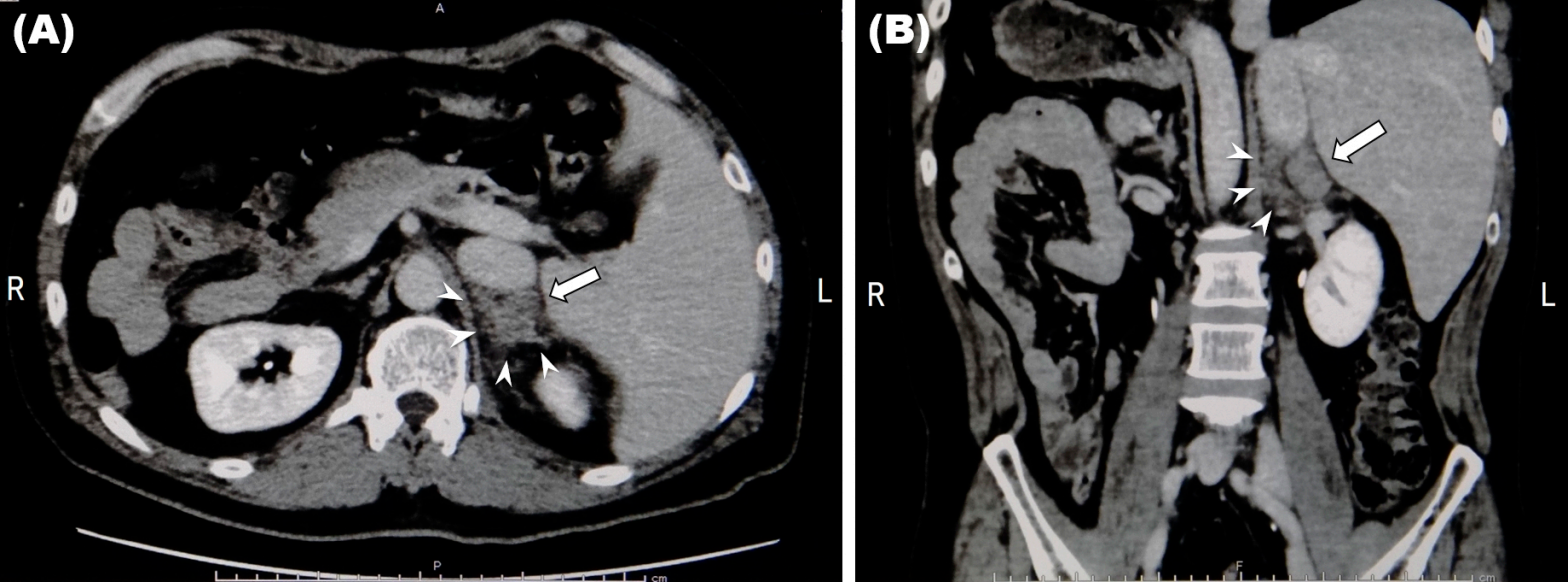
Contrast-enhanced computed tomography showing an enlarged adrenal gland with a 3.1-cm major axis (arrows) and stranding of the periadrenal fat (arrowheads). Other organs are intact. (A): Transverse section. (B): Coronal section.
